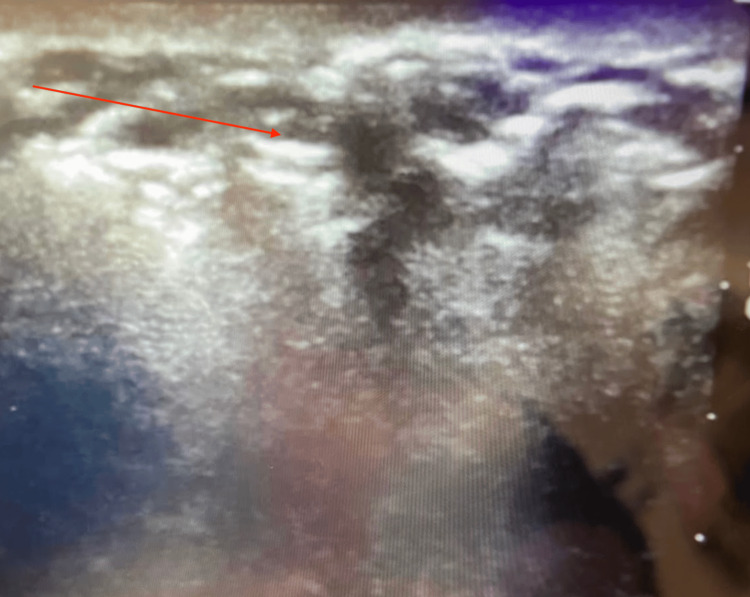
Postoperative, ultrasound-guided TAP block attempt, which revealed obscuration of anatomical landmarks due to extensive subcutaneous air. TAP, transversus abdominis plane
